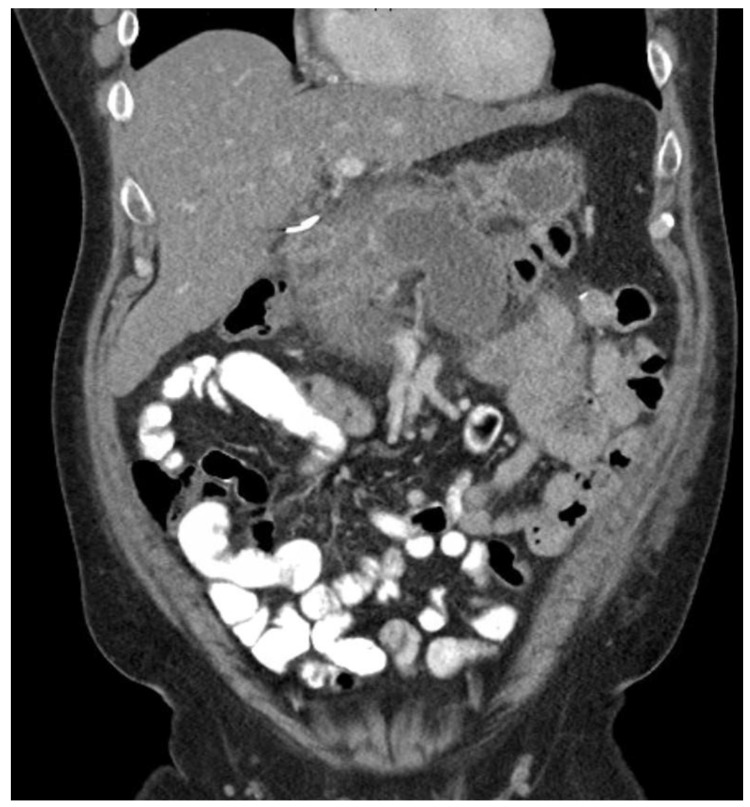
Pancreatitis with CT showing walled-off necrosis.
